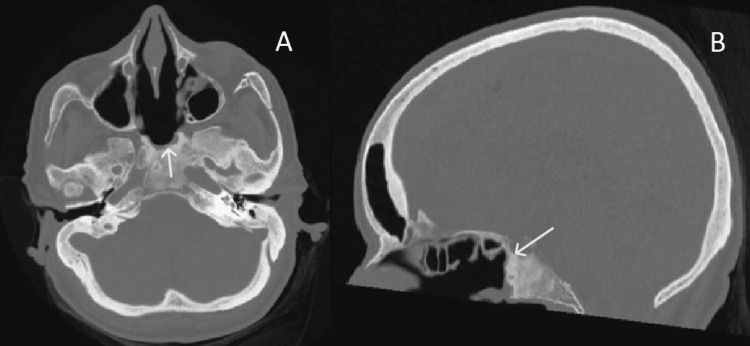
Axial (A) and sagittal (B) bone window CT images of the same patient, after endoscopic surgical approach. There was partial removal of the lesion.
